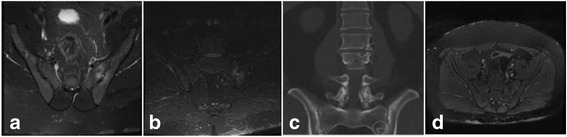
a MRI SIJs (axial) pre-treatment T2 FS demonstrating T2 hyperintense synovitis and marrow oedema b MRI SIJs (coronal) pre-treatment STIR demonstrating acute left sacroiliitis c Conventional CT lumbar spine and SIJs (coronal) with arrows on the L2/3 endplate erosions and circle at the LEFT SIJ erosions d MRI pelvis (axial) post-treatment T2 FS demonstrating resolution of T2 hyperintense synovitis and marrow oedema
